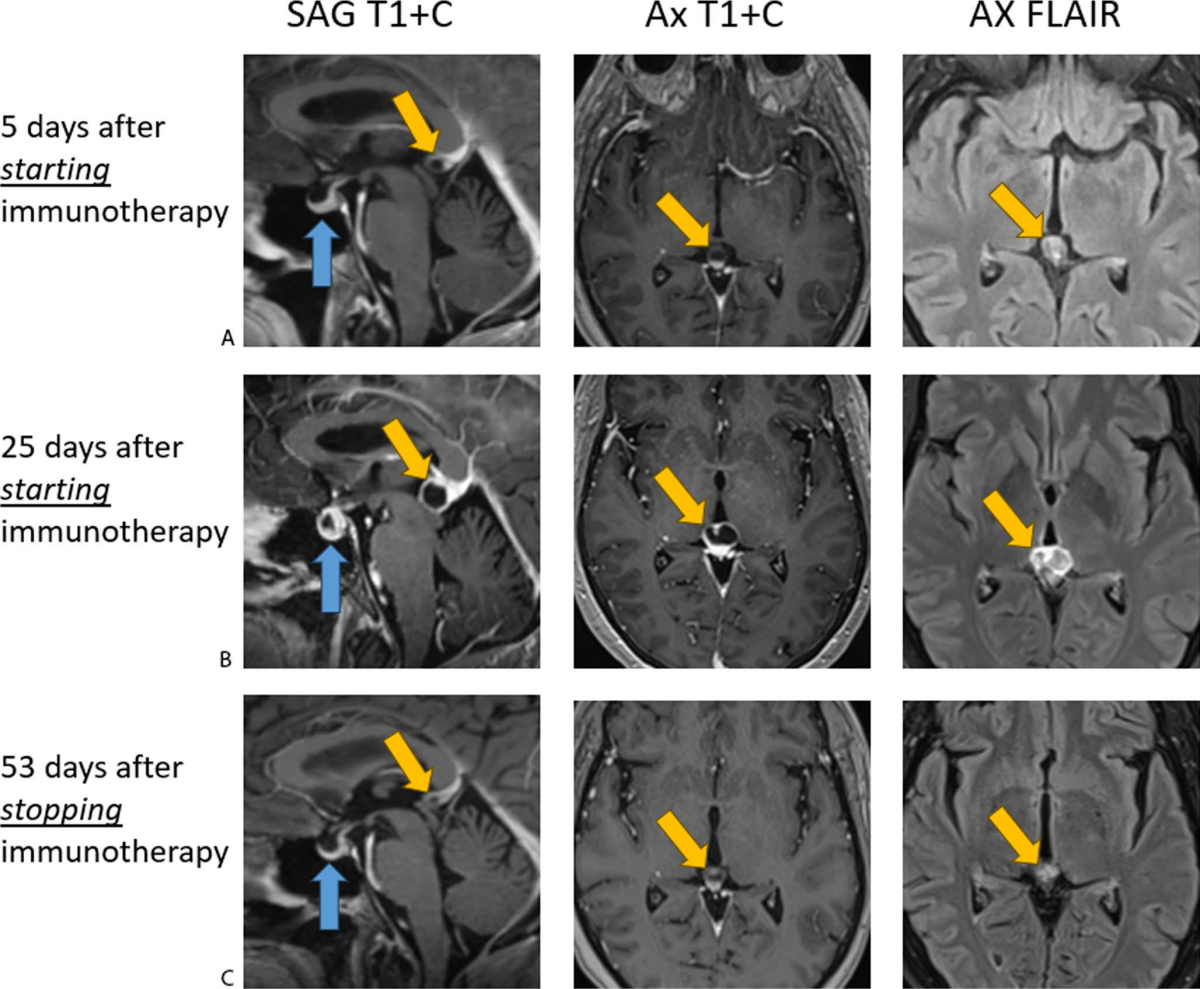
Demonstrates the Sagittal T1 post contrast, Axial T1 post contrast, and axial FLAIR sequences of the brain. A is the baseline demonstrating two tiny, non-enhancing simple pineal cysts with normal pituitary gland. B shows interval increase in size of the pituitary gland and pineal cysts. The pituitary gland was enhancing heterogeneously. There is also increased peripheral enhancement of the pineal cysts. C is 53 days after stopping immunotherapy demonstrating complete resolution of the pituitary and pineal abnormalities
